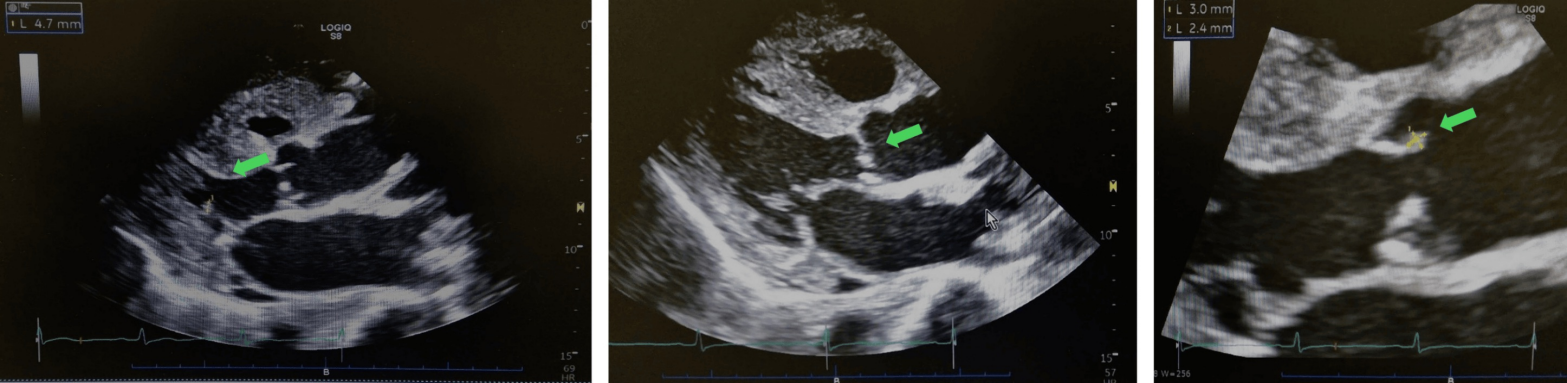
Echocardiograms taken on day 20 (left panel) and day 27 (right two panels). Echocardiogram on day 20 suggested vegetation of the mitral valve (the arrow on the left panel). It disappeared on day 27, but a new vegetation-like mobile mass of the right coronary cusp of the aortic valve was observed (the arrows on the middle and right panels).
